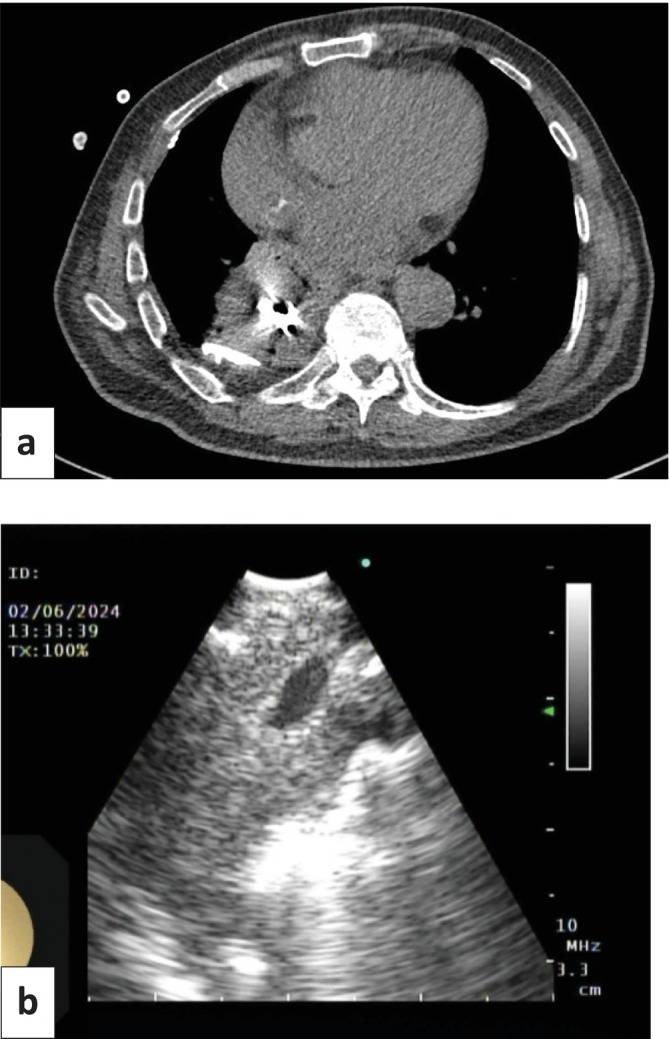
(a) Chest CT redemonstrated 6.8 × 7.2 cm right lower lobe mass with embolization coils in the centre, stable in size compared to 6 months prior. Pleural calcifications and a trace right pleural effusion were also noted. (b) Endobronchial ultrasound image of right lower lobe mass, notable for a vessel coursing through the mass as well as embolization coils, which were avoided during biopsy.
